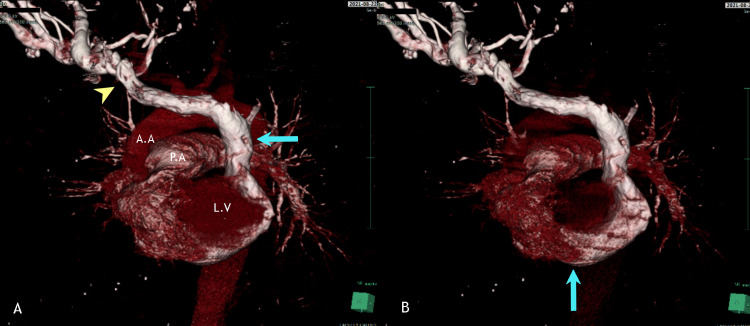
Anterio-lateral view of a 3D reconstruction of CT angiogram in which contrast was injected through the right subclavian vein. 6A: Anterio-lateral view of a 3D reconstruction of CT angiogram in which contrast was injected through the right subclavian vein. The right IJV and right subclavian drain into the right brachiocephalic vein (arrowhead), which then travels to the left side to drain into the isolated PLSVC (arrow). Notice absence of the normal right-sided SVC. 6B: After subtracting the appearance of the Left ventricle and aortic structures, the pathway of the isolated PLSVC is evident as it drains into the dilated coronary sinus (arrow), which follows a route inferio-posteriorly to the left ventricle. LV: left ventricle, AA: ascending aorta, PA: pulmonary artery
